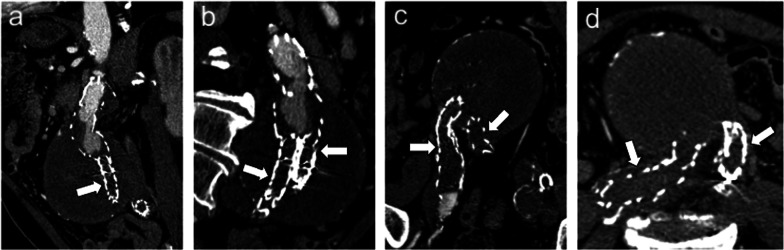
87-year-old man with bilateral iliac stent occlusion. a, b Sagittal and (c, d) CT multiplanar reconstruction images show non-enhancing concentric thrombus inside the lumen of the endograft limbs, with subsequent endograft limb occlusion (arrows)
